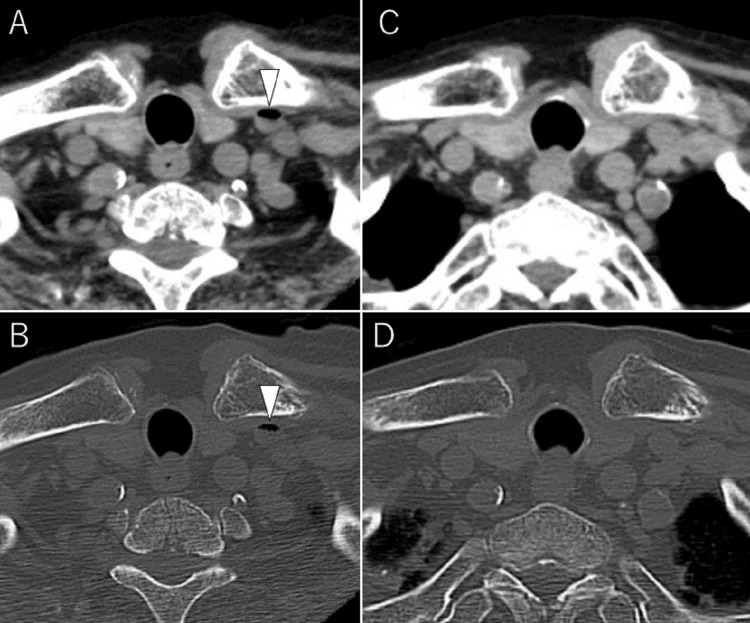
Air in the left subclavian vein on cervical CT. (A) Axial cervical CT image (soft tissue window) showing a small focus of air within the left subclavian vein (arrowhead). (B) Corresponding bone window image confirming the same finding (arrowhead). (C, D) Follow-up CT images at the same level, showing the disappearance of air from the left subclavian vein.
